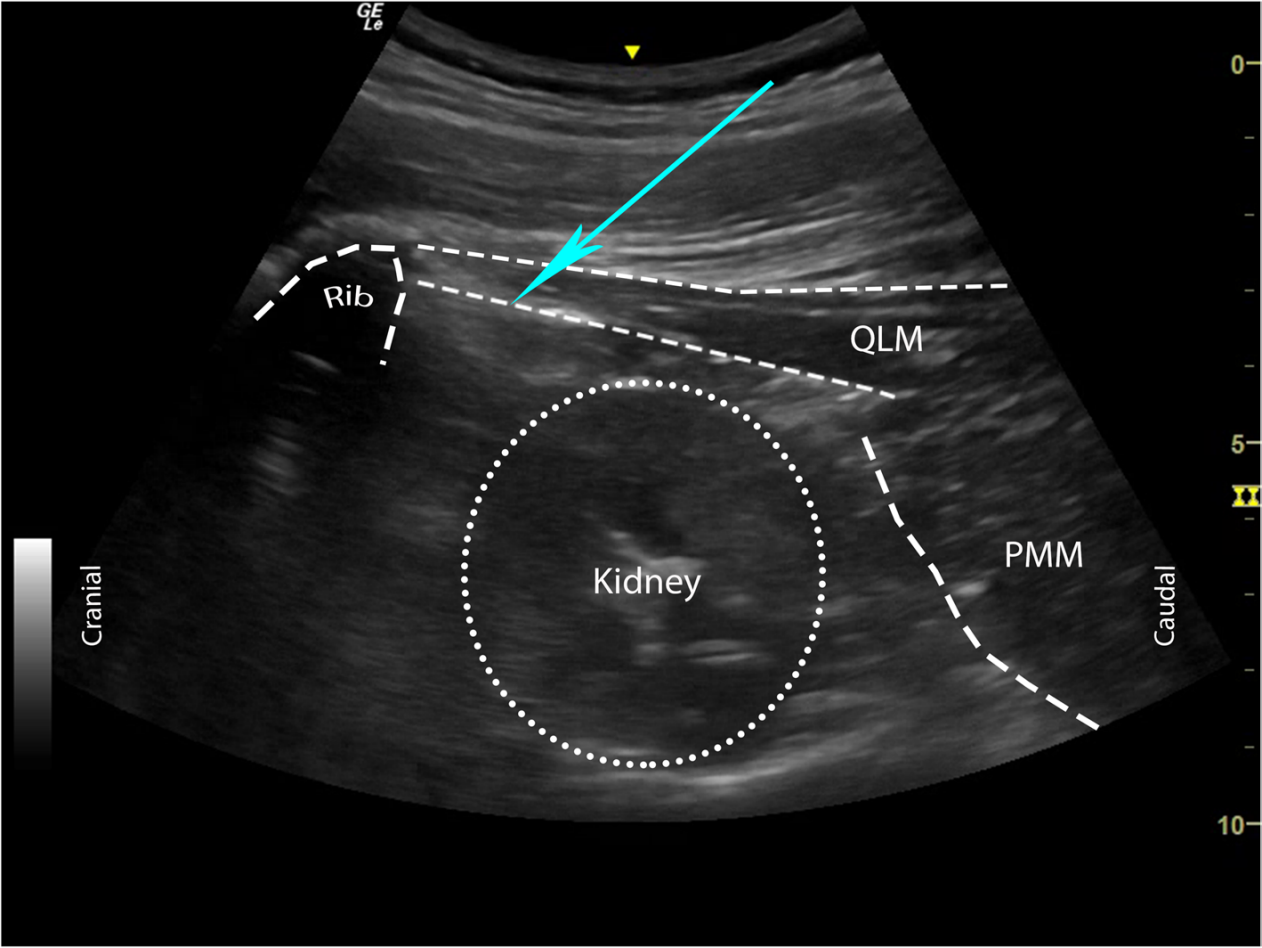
The sonographic landmarks of the modified subcostal QLB3 technique are depicted in an ultrasound image. The arrow indicates caudal-to-cranial needle trajectory. Abbreviations: QLB3, anterior quadratus lumborum block; QLM, quadratus lumborum muscle; PMM, psoas major muscle
